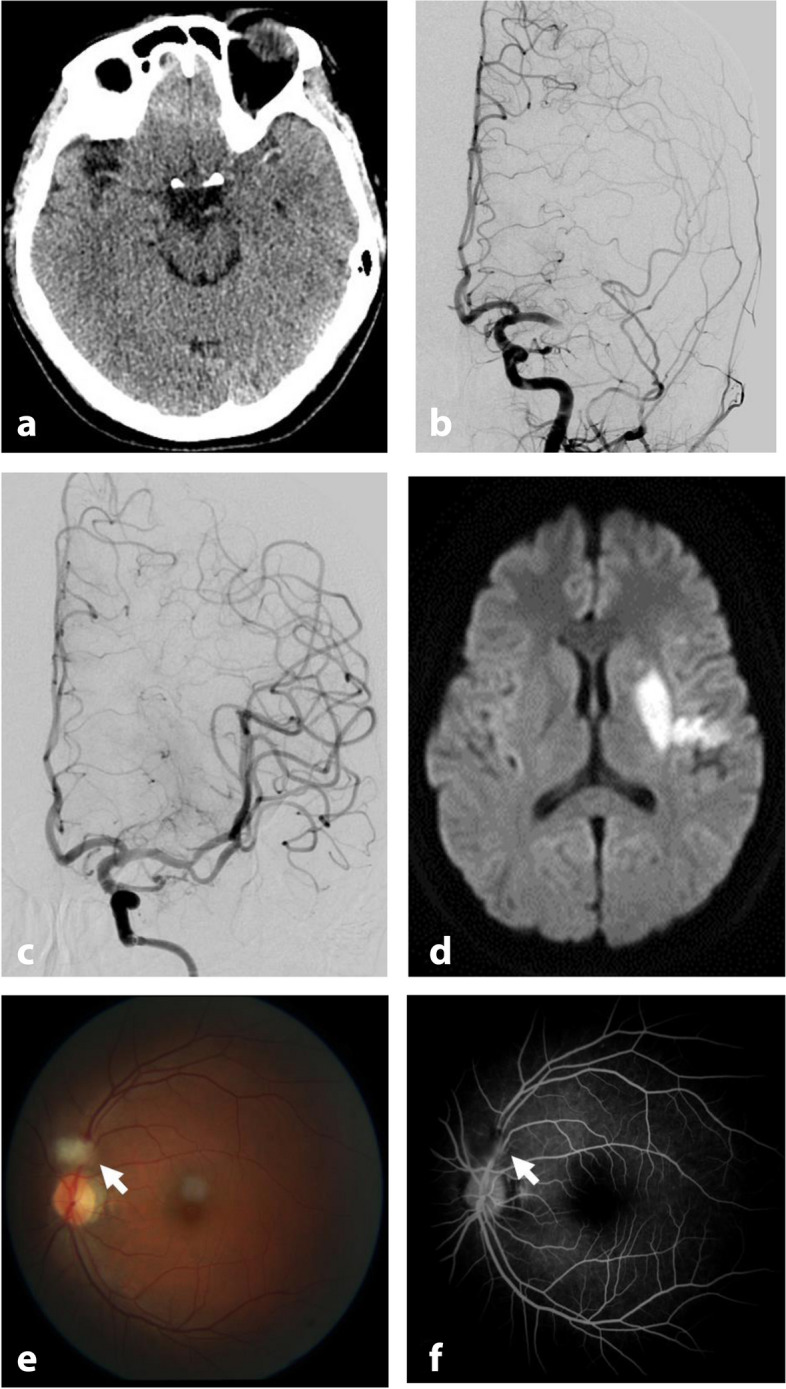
Imaging of vessel occlusion and resolution. a Head computed tomography image showing a hyperdense left middle cerebral artery (MCA) sign. b Diagnostic cerebral angiography demonstrating occlusion of the left MCA M1 segment. c Postoperative cerebral angiography demonstrating reperfusion of the MCA following mechanical thrombectomy. d Diffusion-weighted brain MRI demonstrating lesions in the left basal ganglia. e and f Fundus fluorescein angiography images demonstrating obstructed branches of left retinal artery
